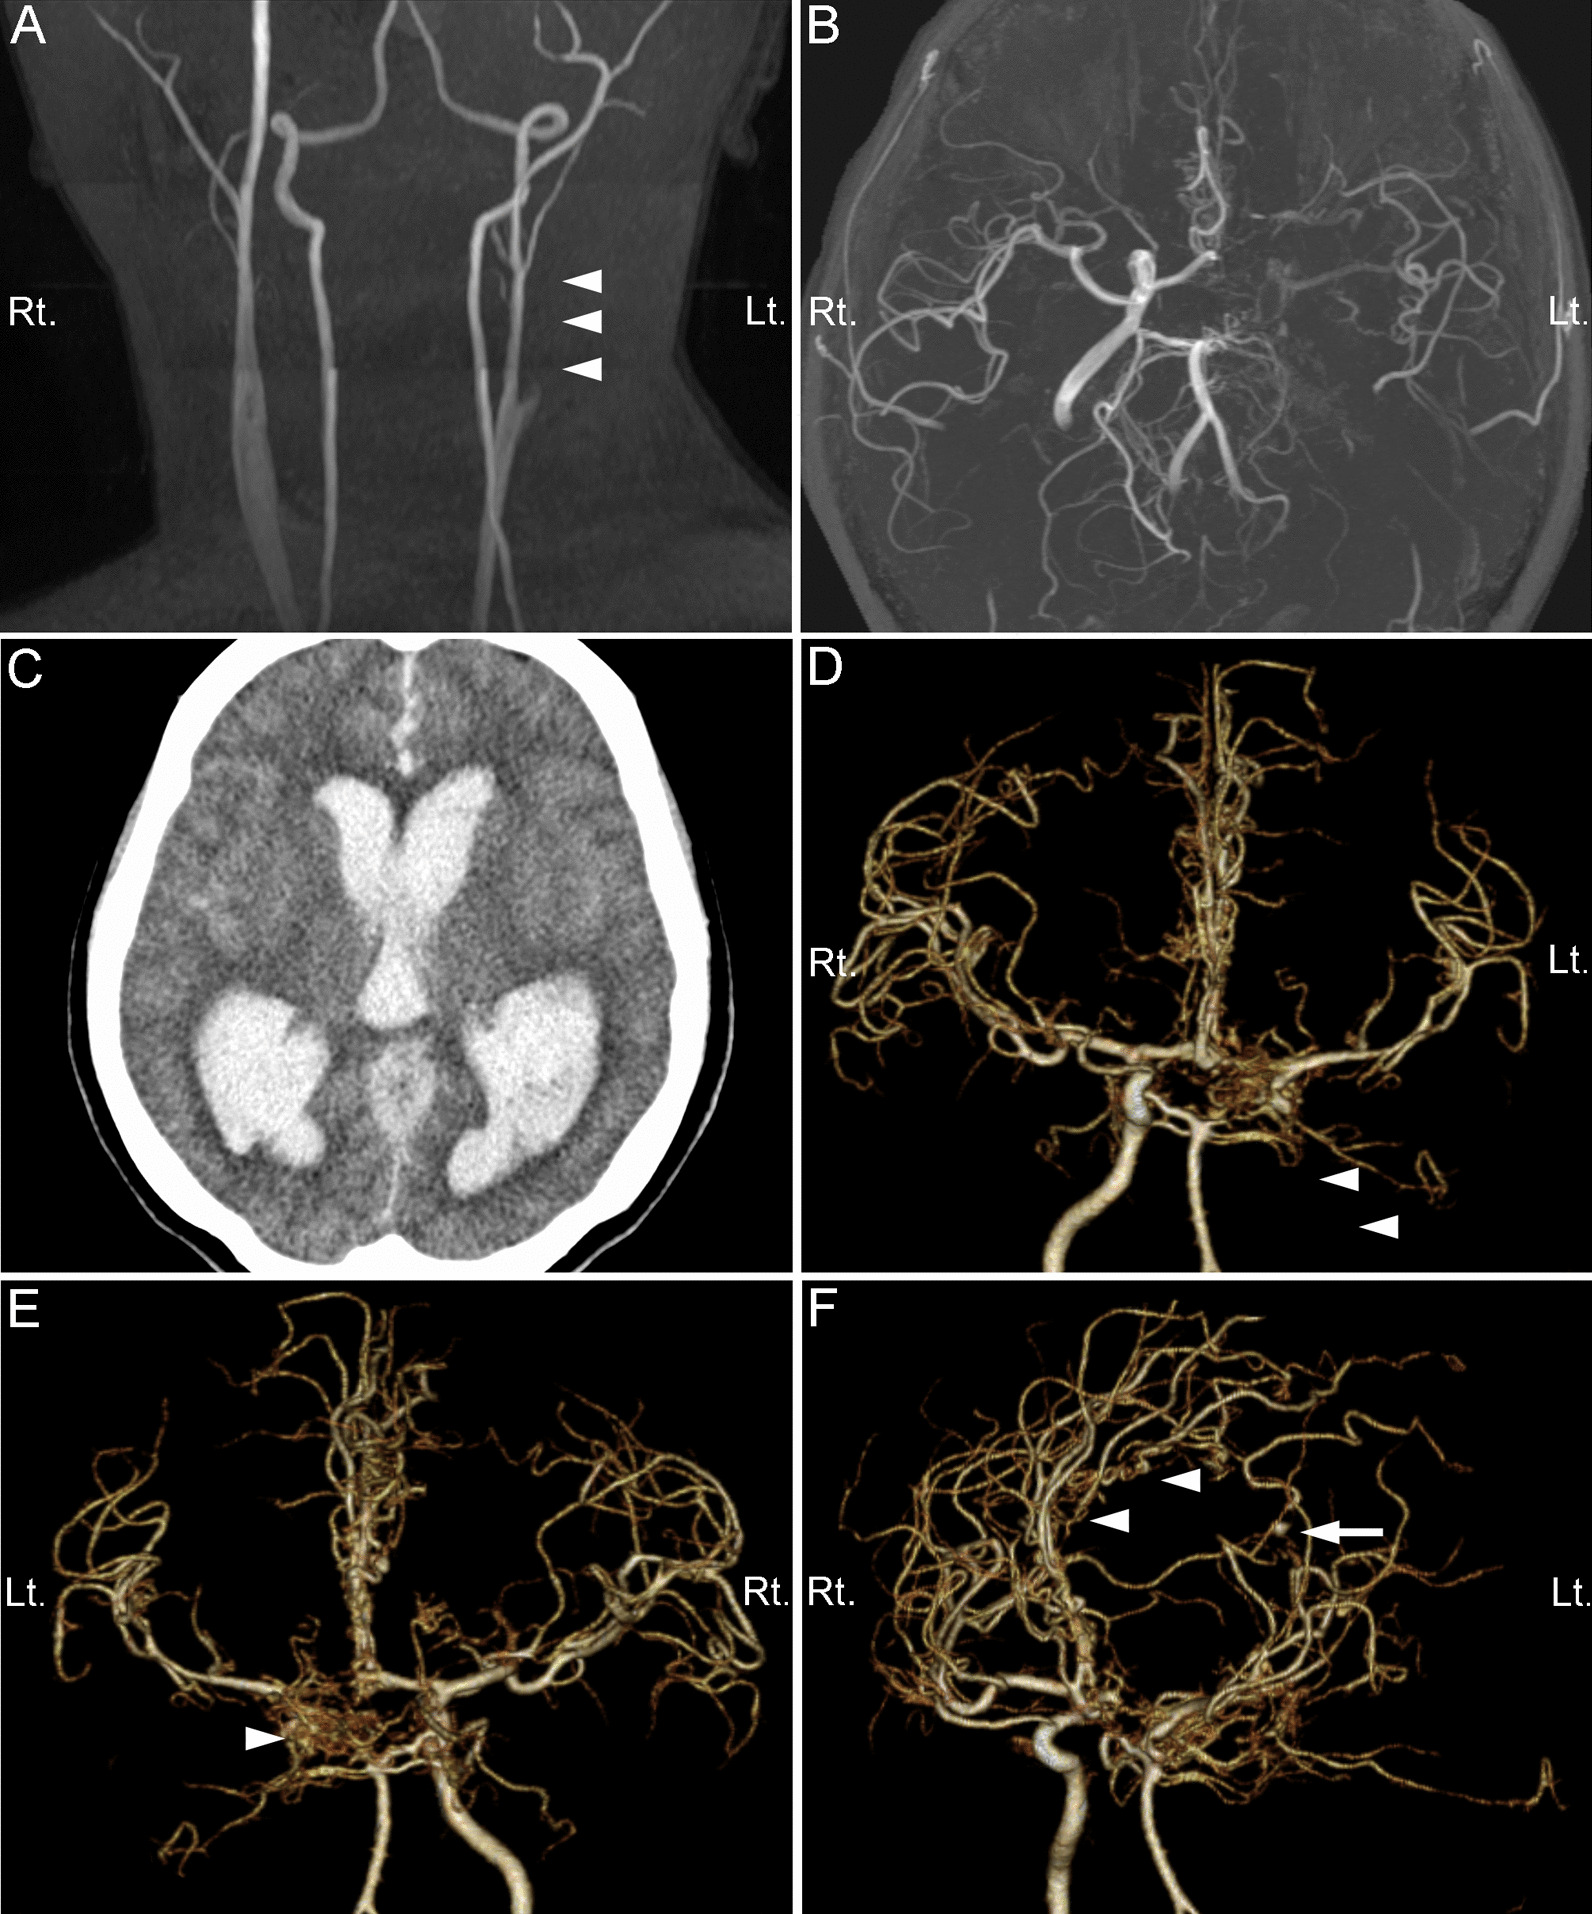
Magnetic resonance imaging of the A neck and B head 6 years before the intracranial hemorrhage described in this report. The diagnosis at that time was obliteration of the left internal carotid artery (ICA) (A; arrowheads) with unilateral moyamoya disease (B). C Preoperative plain computed tomography (CT) of the brain parenchyma window. D–F Three-dimensional CT angiography (3D–CTA) images showing anteroposterior (D), posteroanterior E, and left anterior oblique 45° F views. The three-dimensional CT angiography images show obliteration of the left ICA (D; arrowheads), rete mirabile of the left posterior communicating artery and left posterior cerebral artery (E; arrowhead), rete mirabile of the left anterior cerebral artery (ACA; F; arrowheads), and a distal ACA aneurysm (F; arrow). *Lt.* left; *Rt.* right
